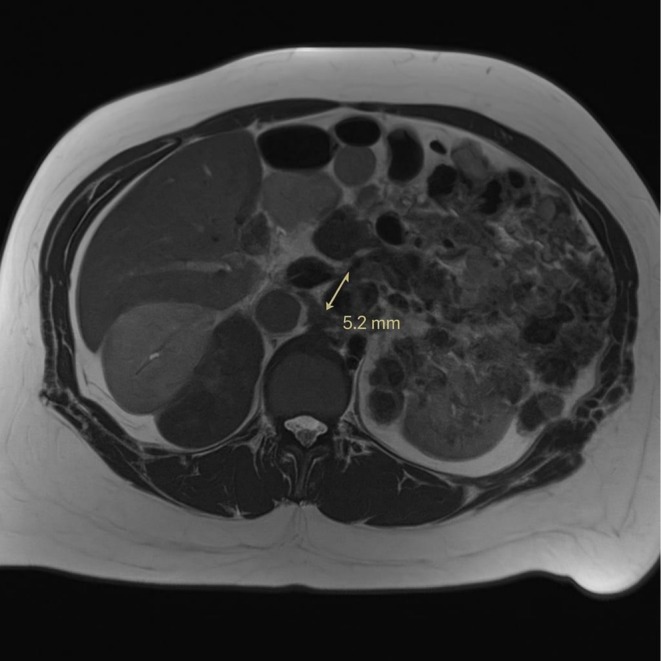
Contrast‐enhanced axial CT scan of the abdomen: A rounded, well‐defined, low attenuation fluid collection in the left upper quadrant, consistent with peripancreatic fluid collection.
